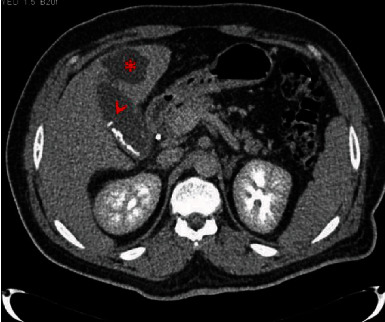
Abdominal CT scan showing gallbladder containing stones (arrow), with nearby segment IV b abscess (asterisk).
